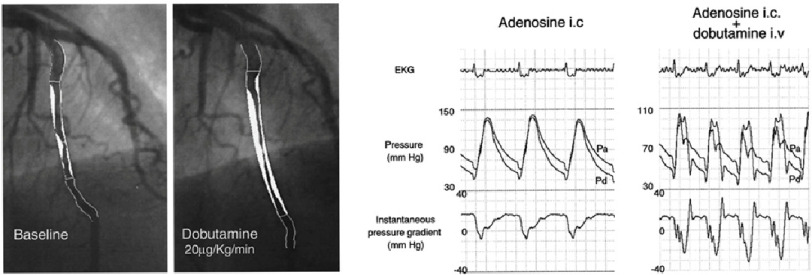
X-ray angiogram showing coronary artery morphology change under dobutamine stress (left) and intravascular pressure measurement across MB section under dobutamine and adenosine stress (right), reported by Escaned et al.^46^. Pa, aortic pressure; Pd, intracoronary pressure distal to the MB.
